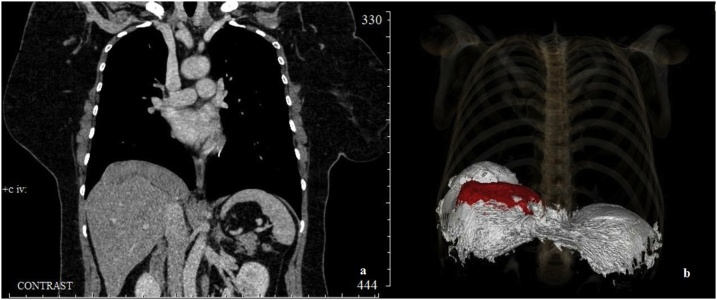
Chest CT coronal scan with 3D CT volume rendering picture of the tumor and the diaphragm.
